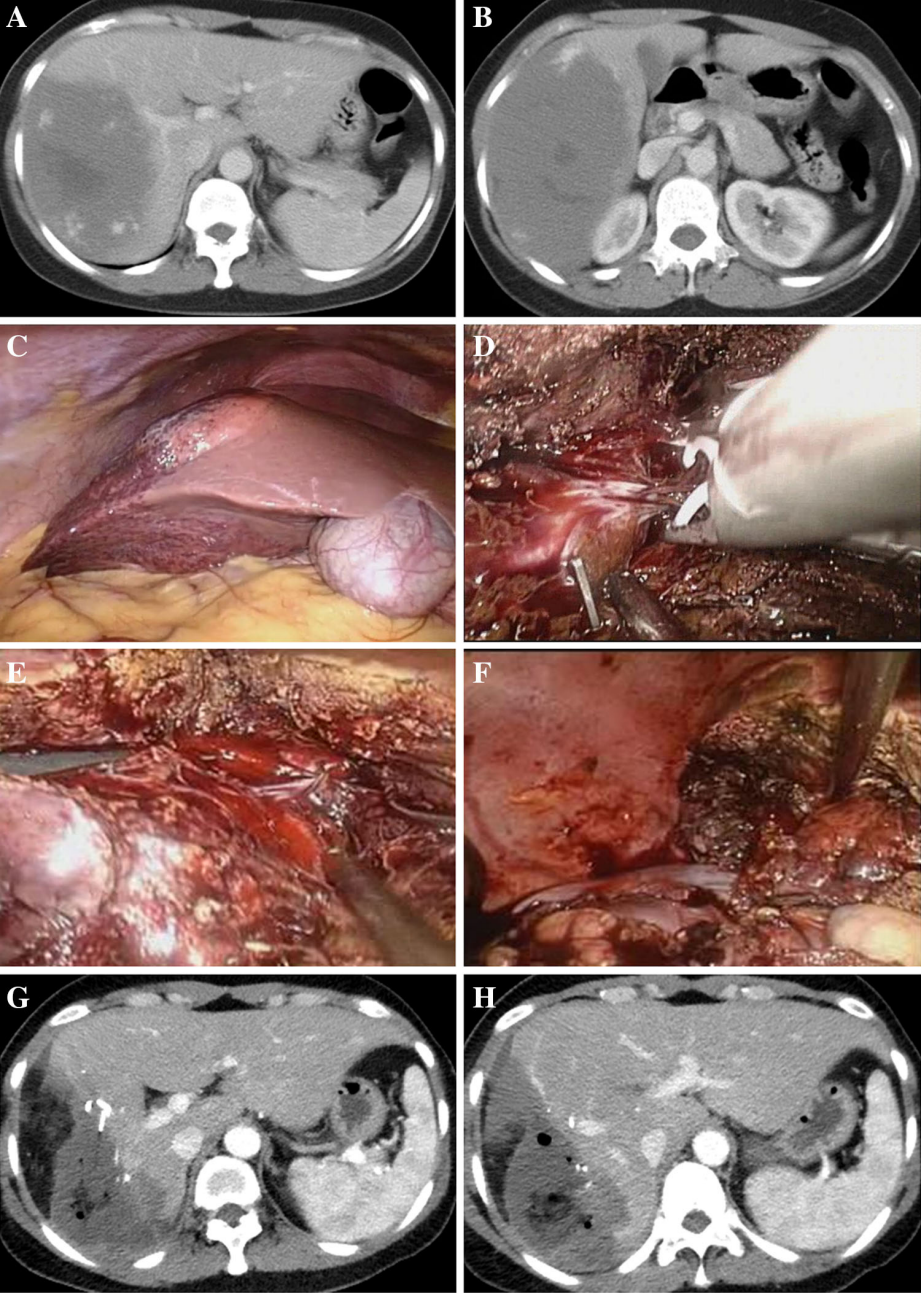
Successfully performed enucleation for giant liver hemangiomas with tumor diameter of 21.7 cm in the right posterior segment and reduced excessive loss of normal liver tissue. A, B typical features of hemangioma with contrast-enhanced CT; C tumor exterior characteristics with a laparoscopic horizon; D branches of middle the hepatic vein ligate with Hem-o-lok; E enucleation of hemangiomas along the tumor margin; F the cut surface of the remnant liver; G postoperative contrast-enhanced CT of tumor enucleation without excessive loss of normal liver tissue; H Retained right anterior portal branches without injury
